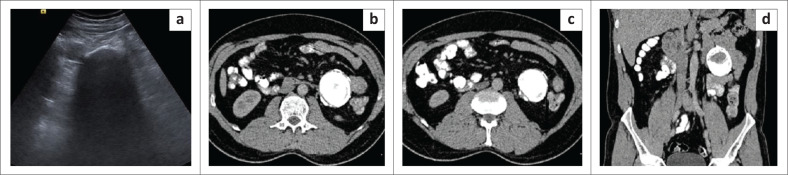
Calcifying fibrous tumour of the mesentery. Transverse grey scale ultrasound image (a) reveals a calcified lesion in left lumbar region causing extensive posterior acoustic shadowing. Axial contrast enhanced CT images (b, c) and coronal reformatted contrast enhanced CT image (d) reveal a well-defined oval smooth marginated mass lesion in the mesentery with near complete calcification, abutting the bowel loops.
